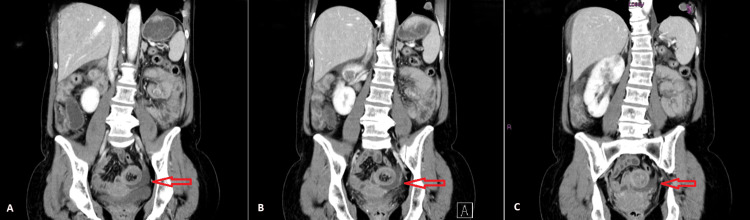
CT scan showing the probable location of the ileo-ileal intussusception with associated free fluid (A-C) and bowel wall thickening (B), and CT findings of intestinal distress. A small residual mass consistent with previous metastasis can also be noted on the inferior lobe of the left lung.
